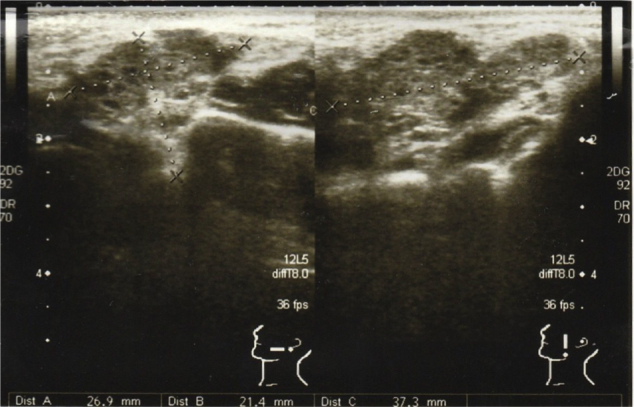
Ultrasound of left cheek showed a mass of 26.9 × 21.4 × 37.3 mm.
